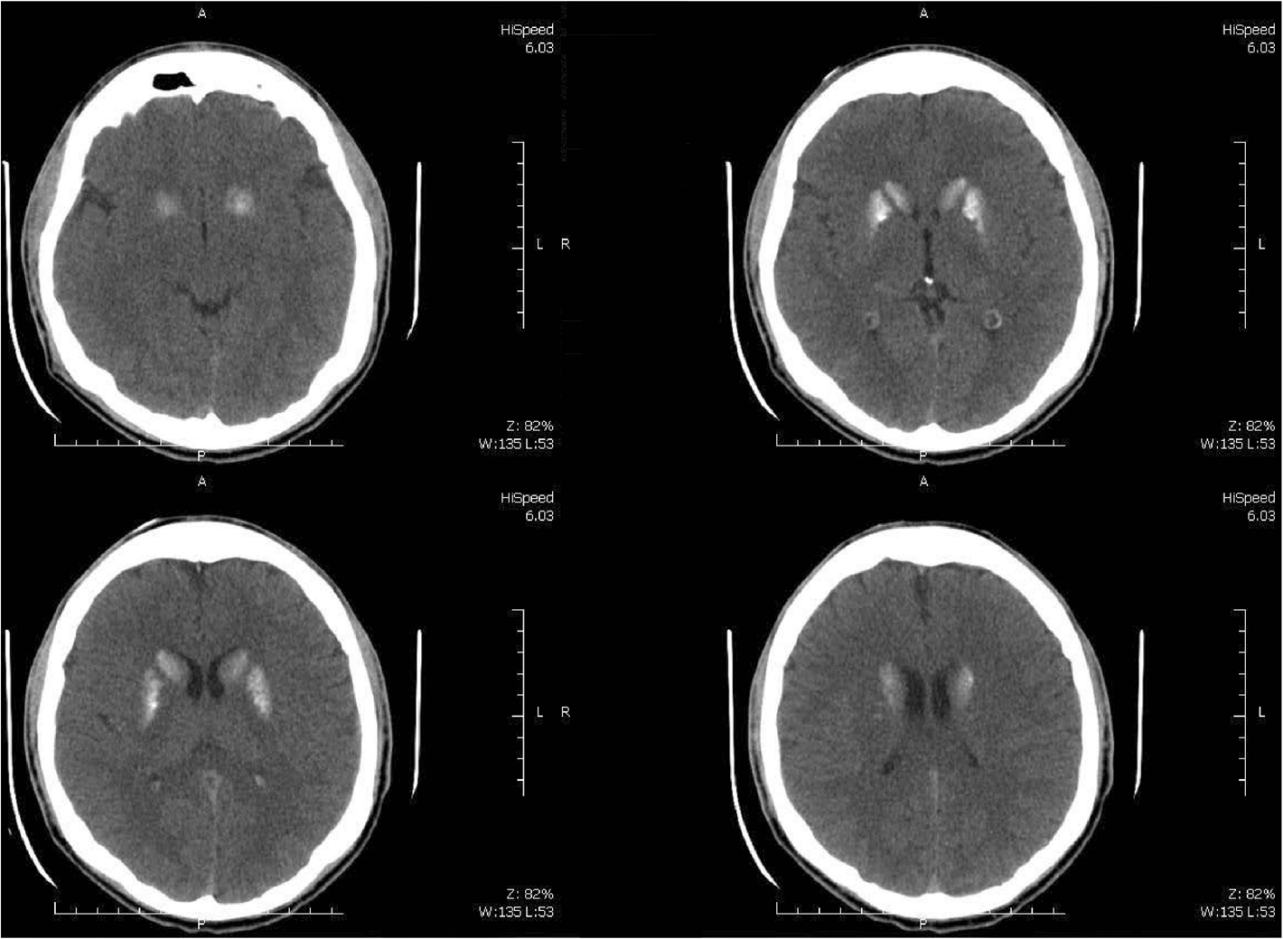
Computed tomography scan of the brain.
